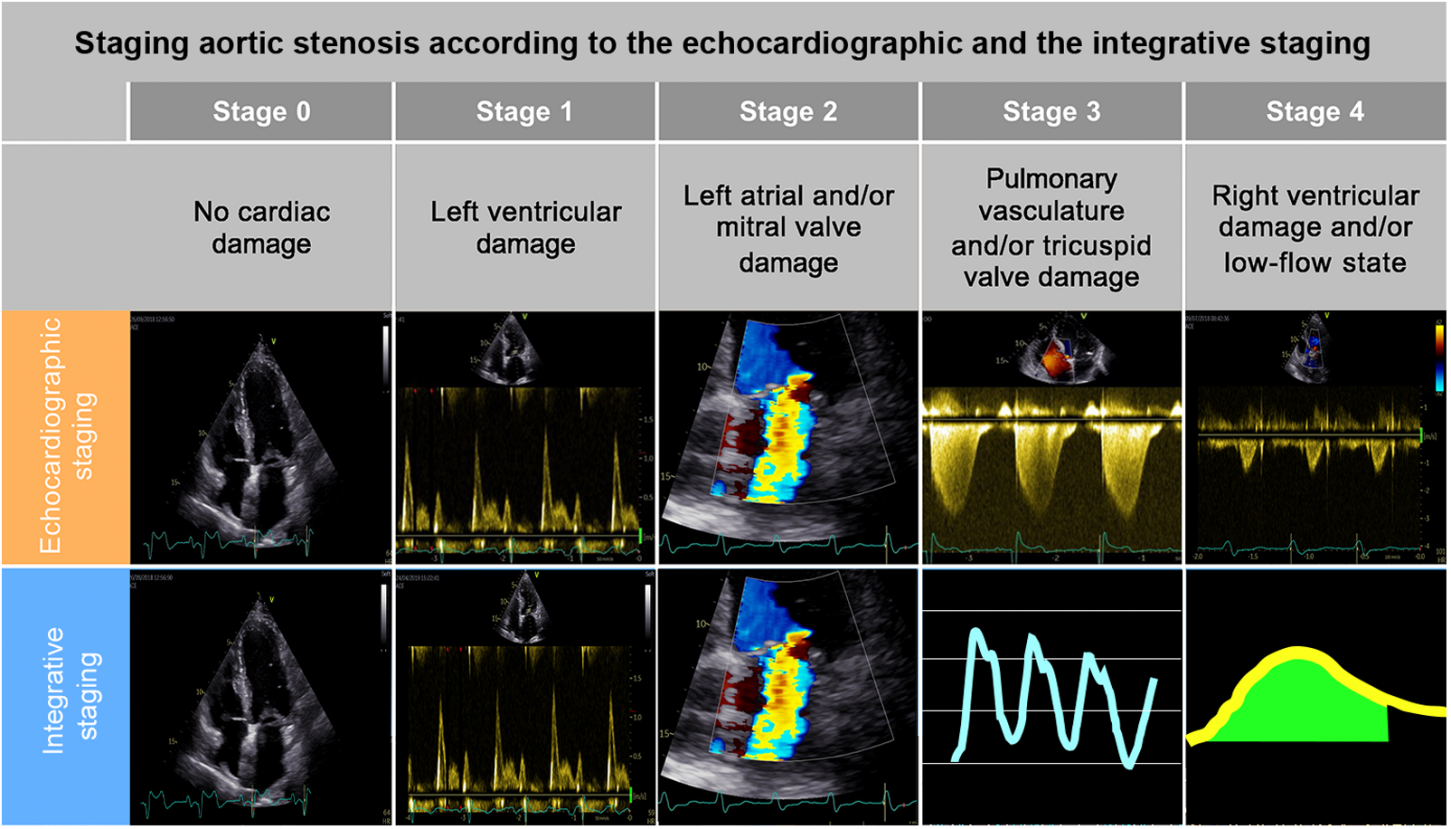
Classification according to the two analyzed aortic stenosis staging models and their application in survival probability. A comparison between the main parameters included in the current echocardiographic AS staging (top): stage 0: normal left and right chambers (no cardiac damage); stage 1: restrictive mitral inflow pattern (LV diastolic dysfunction grade 3) with a pathological L-wave during diastasis (LV damage); stage 2: moderate mitral regurgitation (mitral valve damage); stage 3: continuous wave Doppler signal used to calculate RV systolic pressure from the peak velocity of the tricuspid regurgitant jet according to the simplified Bernoulli equation (pulmonary vasculature damage); stage 4: pulsed wave Doppler in LV outflow tract to estimate LV stroke volume (low-flow state) and the proposed integrative staging model (bottom): stages 0,1, 2 as described above, stage 3: pulmonary artery wave pressure tracings during RHC (pulmonary vasculature damage), stage 4: thermodilution method to measure cardiac output during RHC (low-flow state).
